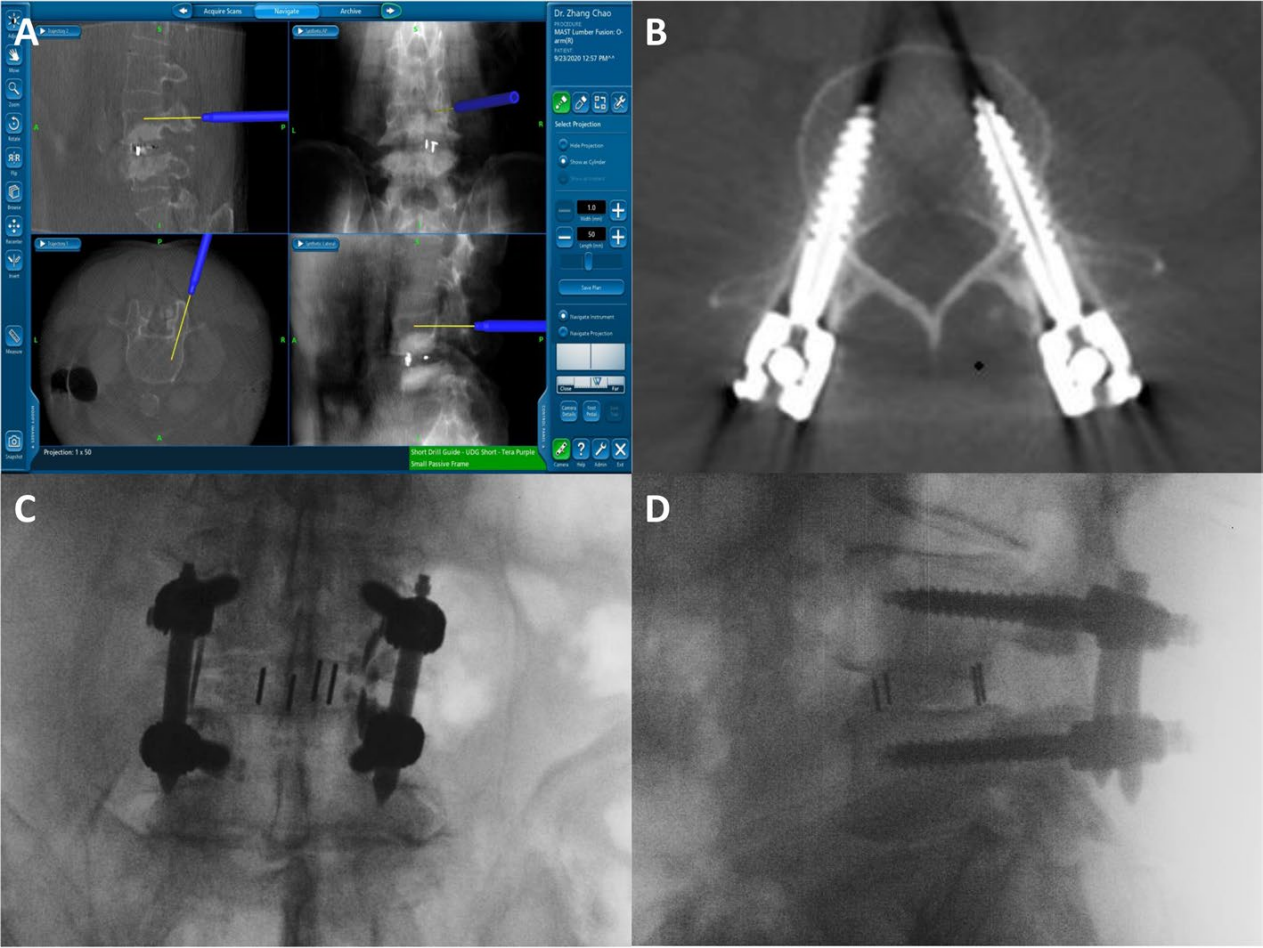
Intraoperative planning and outcomes. A A screenshot of the intraoperative navigation plan, showing the sagittal and axial views of the planned insertion trajectory and the positive and lateral views of the reconstructed fluoroscopy. B Postoperative CT reconstruction showing that the pedicle screws are completely within the vertebral pedicle. C A typical anterior view of a navigation-guided pedicle screw implant. D A typical lateral view of a navigation-guided pedicle screw implant
